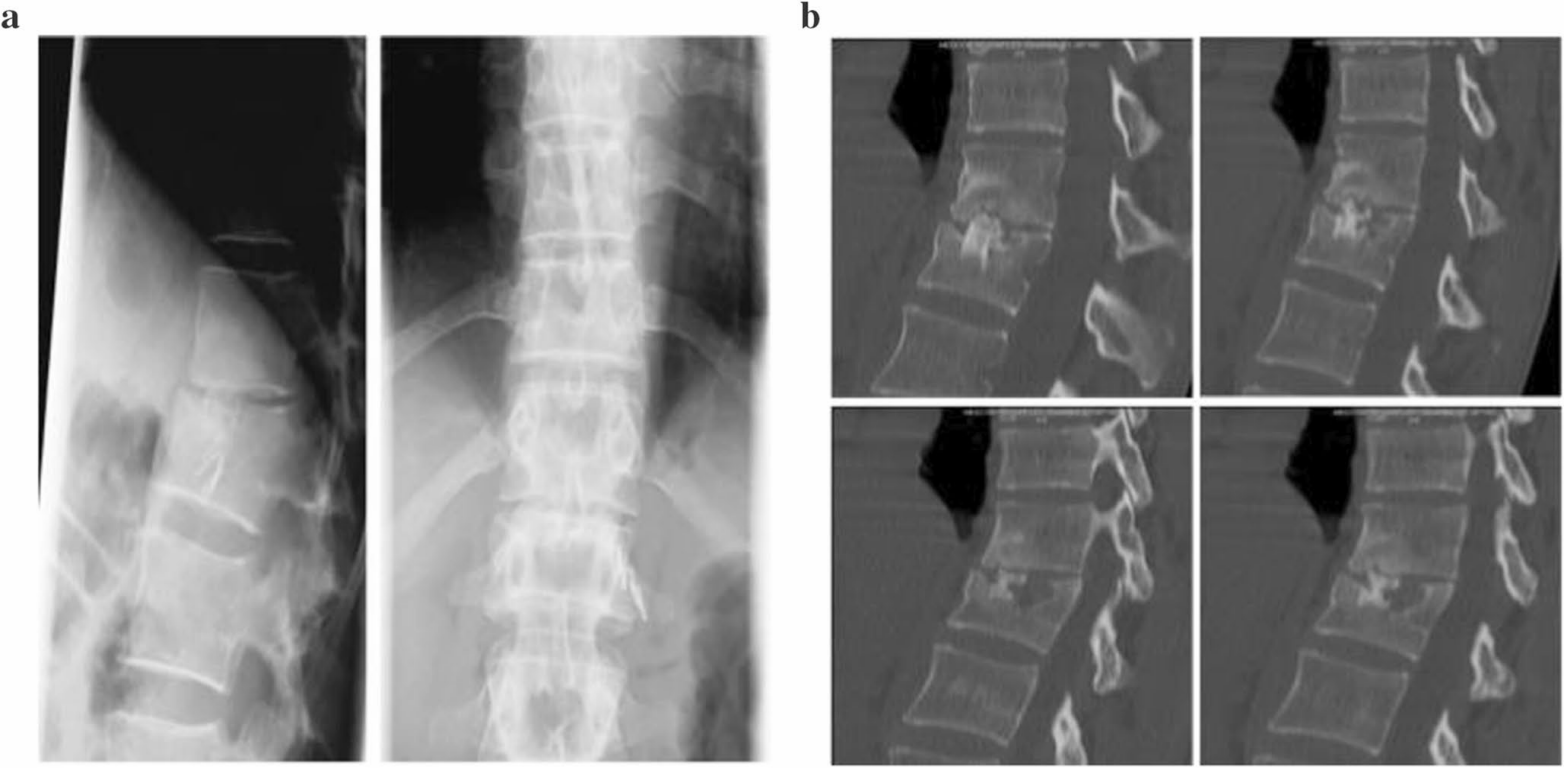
a female, 36y, L1-Fracture type B. No signs of non-fusion in plain radiograph 38 months after surgery b CT scan of the same patient reveals non-fusion in the cranial interface and partial lysis of the graft
